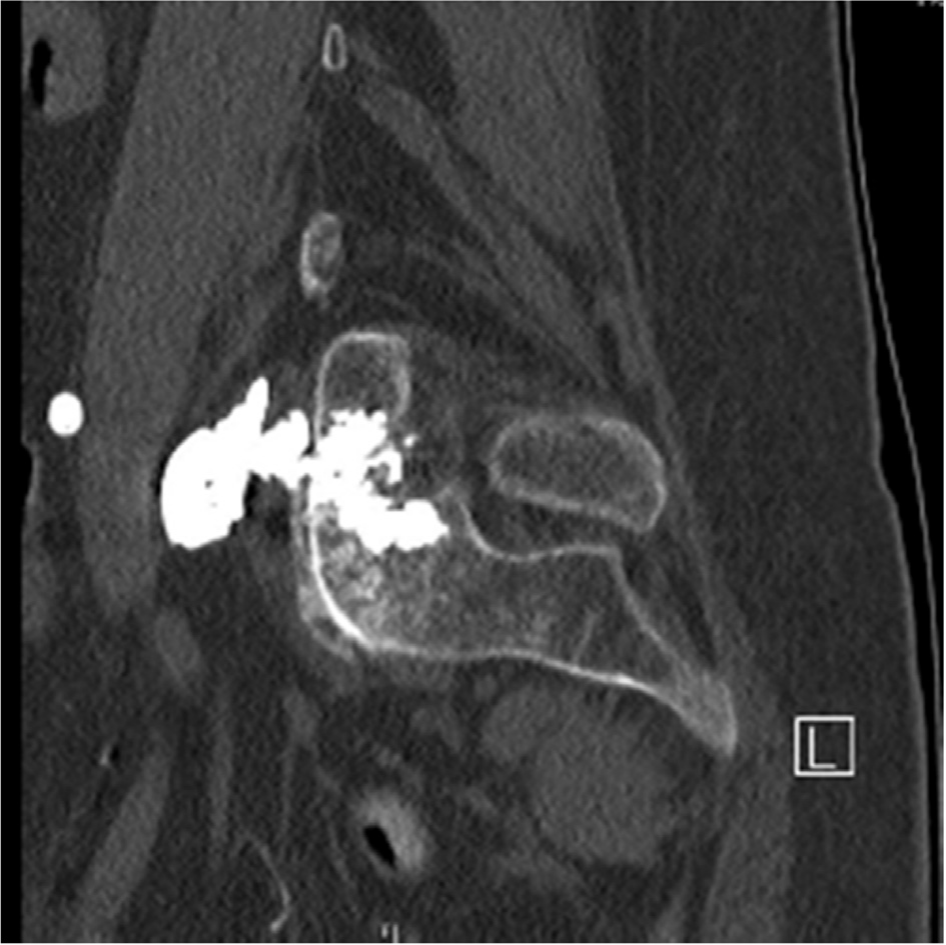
Sagittal view CT without contrast of lumbosacral spine demonstrating cement extravasation extending from the sacral ala posteriorly and abutting the iliopsoas muscle anteriorly.
